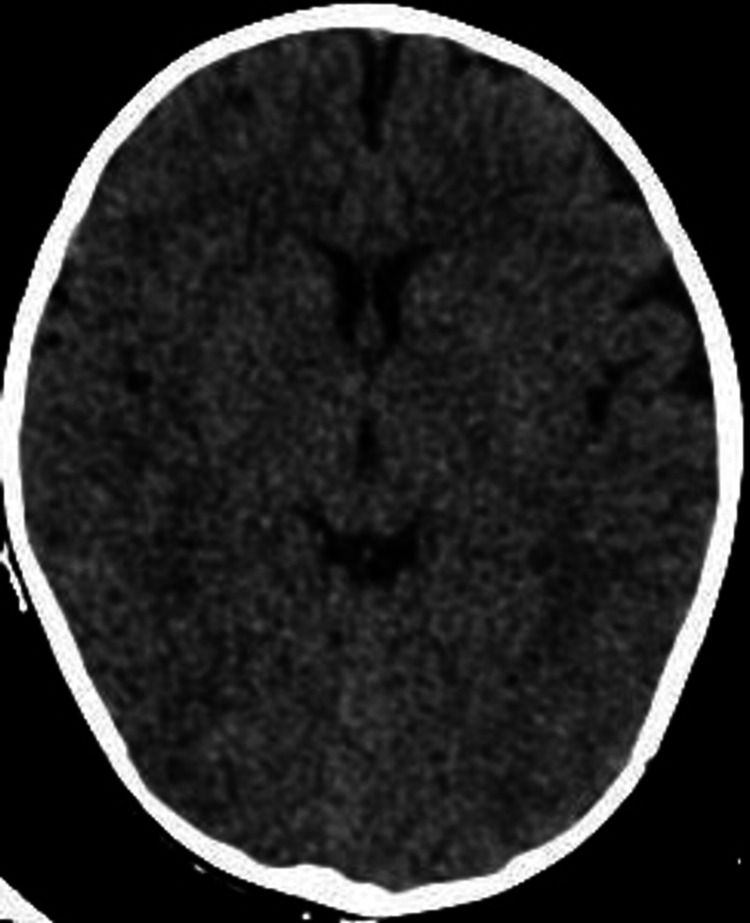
Axial view of the brain CT scan showing normal anatomical findings. CT: computed tomography
